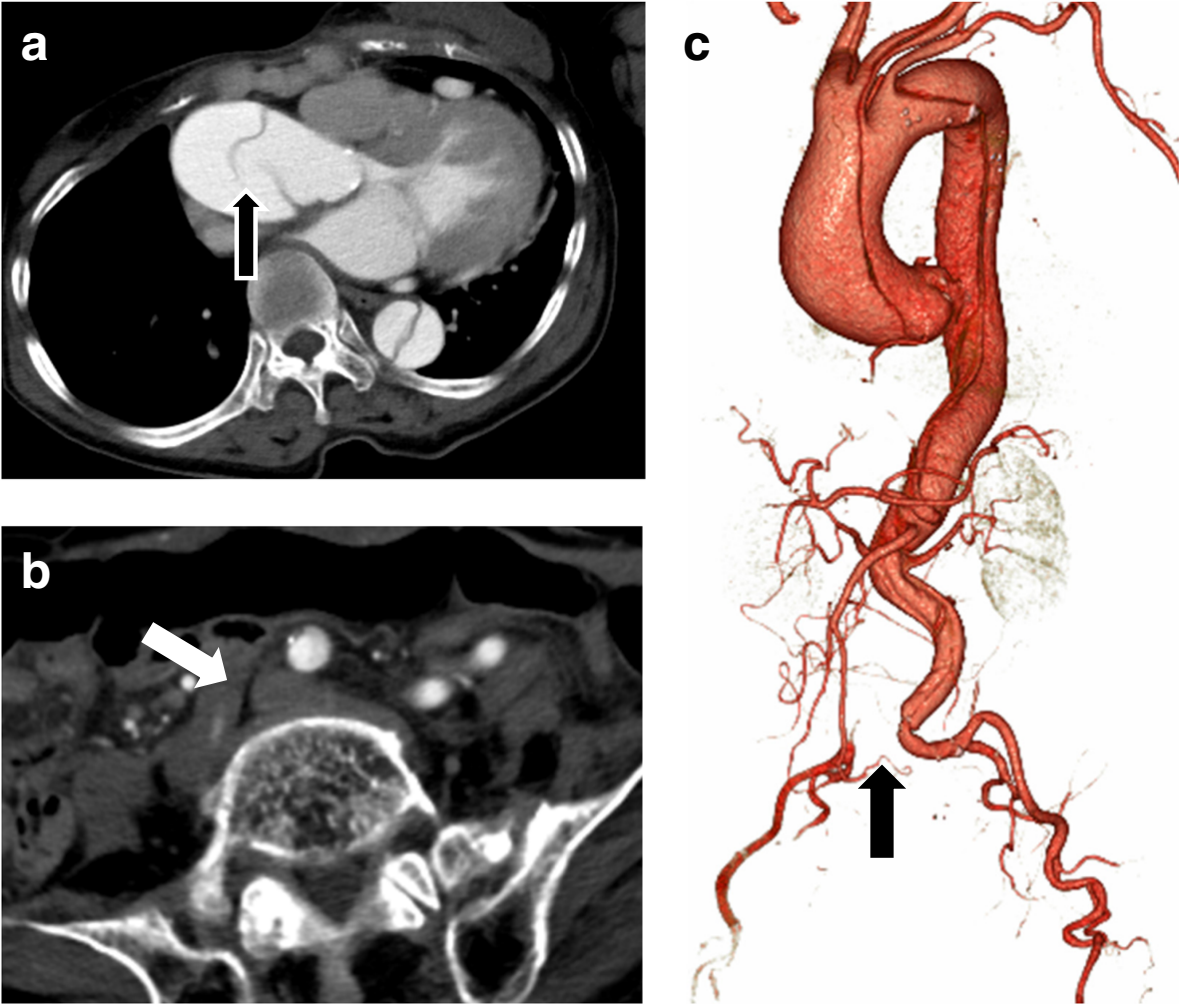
A preoperative CT scan showing acute type A aortic dissection. The dissection entry was found in the ascending aorta (a arrow). The true lumen of the right common iliac artery was compressed by the thrombosed false lumen (b, c arrow)
